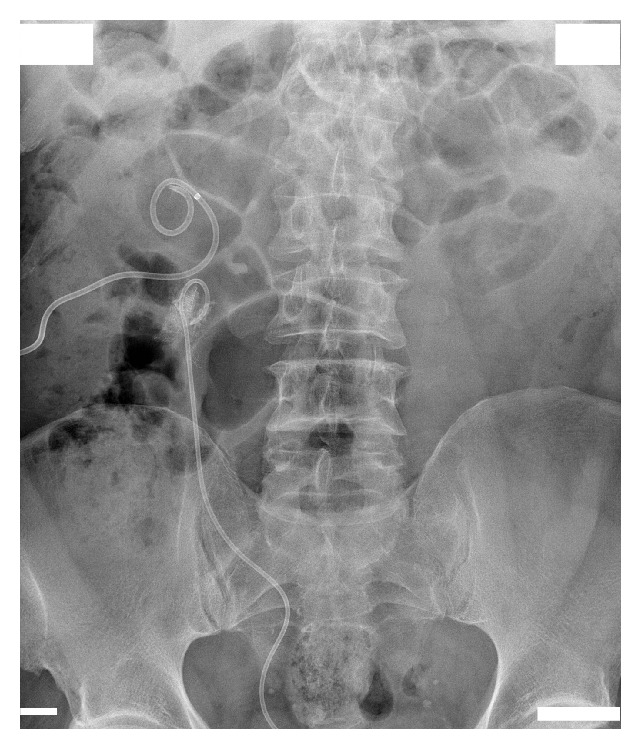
Plain X-ray image of the urinary tract after the semirigid ureterorenoscopy procedure shows that almost half of the mesh stent and its incrustations were completely disintegrated by laser energy.
